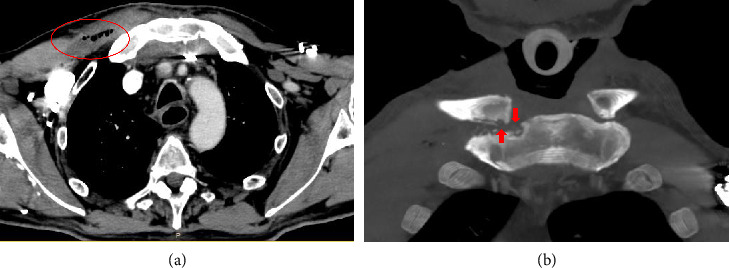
CT scan at 3 weeks: (a) liquid collection containing gas (red circle) in the right pectoralis major. (b) Both clavicle and sternum bone erosions (red arrows).
